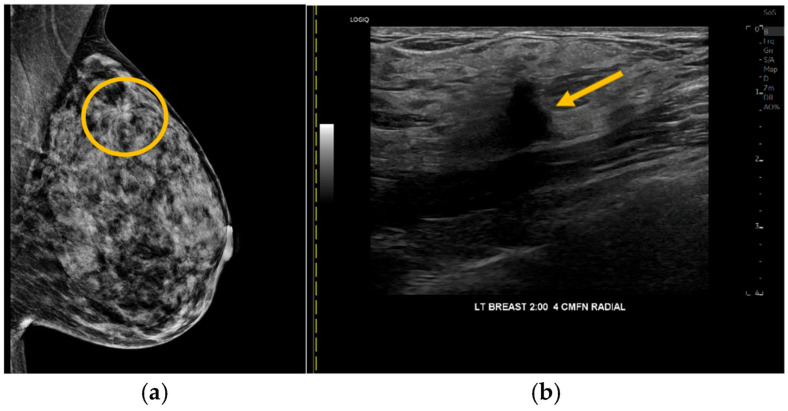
Breast cancer with positive CBE, iBE, and imaging. (a) Mammography demonstrates in the left upper outer breast, posterior depth, a partially obscured mass (circle) with associated architectural distortion. (b) Ultrasound demonstrates a correlating irregular 1.2 cm hypoechoic mass (arrow) biopsied under ultrasound guidance yielding invasive ductal carcinoma.
